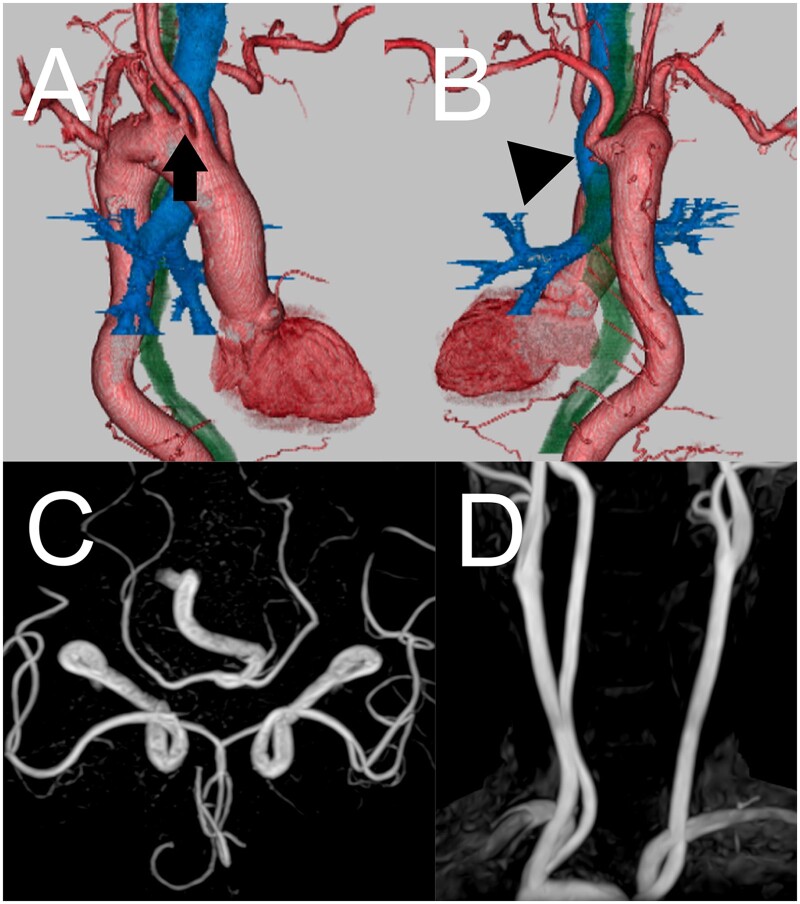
Preoperative three-dimensional computed tomography [(A) right lateral view; (B) posterior view] shows the steep right-sided aortic arch, the Kommerell diverticulum (black arrowhead) of the aberrant left subclavian artery and anomalous right vertebral artery originating from the aortic arch (black arrow). Preoperative magnetic resonance angiography shows the incomplete circle of Willis due to the absence of the left and right posterior communication arteries (C). Magnetic resonance angiography also reveals that the left vertebral artery is missing (D).
